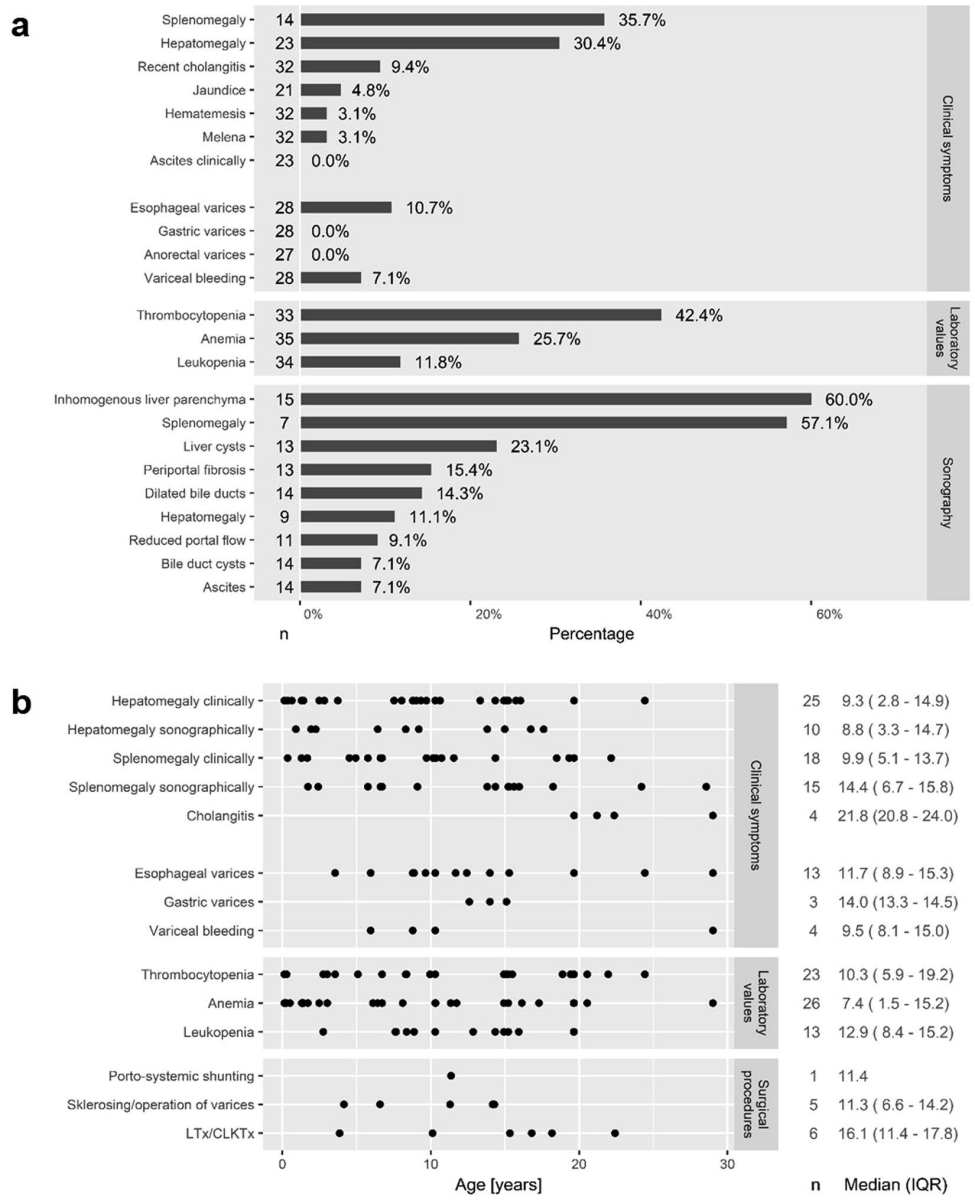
(a) Liver-related clinical symptoms and sonographic abnormalities of 39 adult patients without previous LTx/CLKTx at time of cross-sectional analysis; n = number of informative cases. (b) Dot plot of age at first documentation of signs of portal hypertension and/or liver complications with median and IQR. Events after LTx/CLKTx were excluded; n = number of informative cases.
